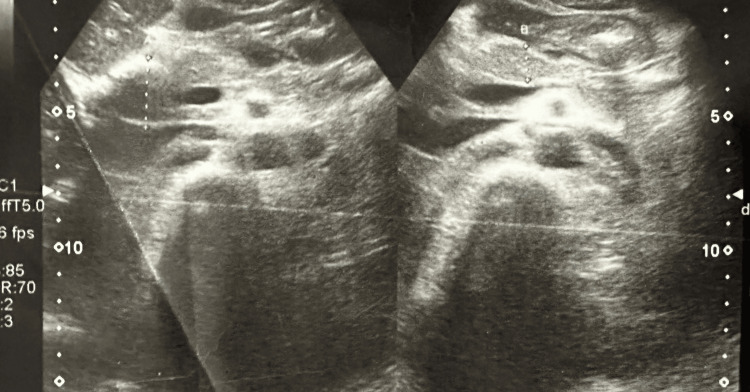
Ultrasound evidence of pheochromocytoma.
